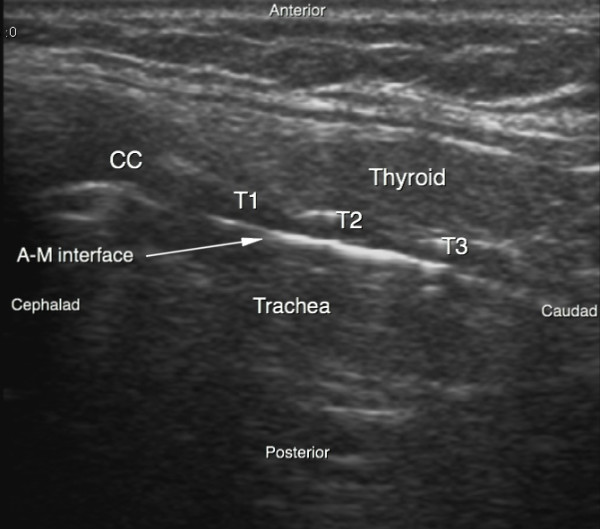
Ultrasound sagittal view of the neck. A-M interface, air-mucosa interface; CC, cricoid cartilage; T1, first tracheal ring; T2, second tracheal ring; T3, third tracheal ring.
